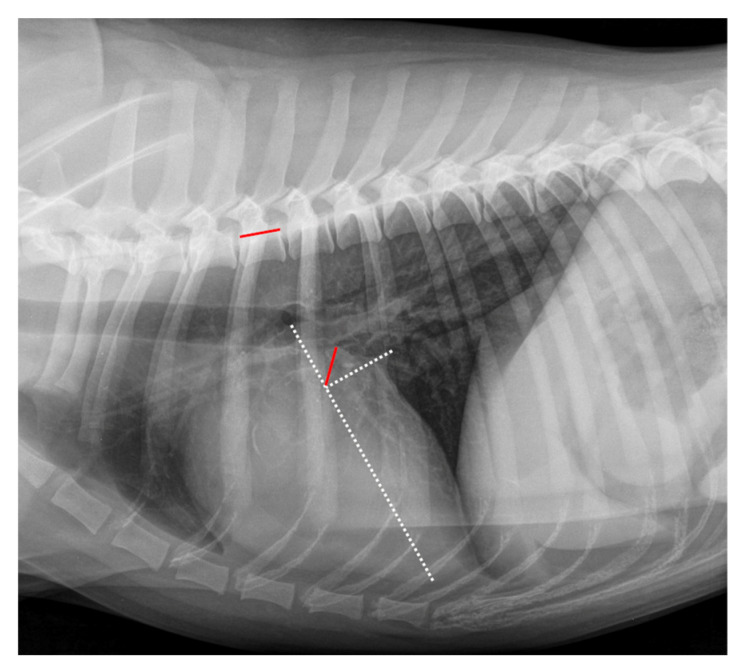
Radiographic Left Atrial Dimension (RLAD) measurement in the same right lateral thoracic radiograph seen in Figure 1. The long axis (white dotted line) was applied as described for the VHS measurement (Figure 1). The short axis (white dotted line) was drawn from dorsal intersection of the caudal vena cava and the cardiac silhouette to the long axis. The bisecting RLAD line was drawn from the intersection point to the dorsal margin of the left atrium (red line on cardiac silhouette). This line was transposed onto the vertebral column (red line on vertebral) as described in Figure 1. The RLAD was 0.8 vertebral units.
